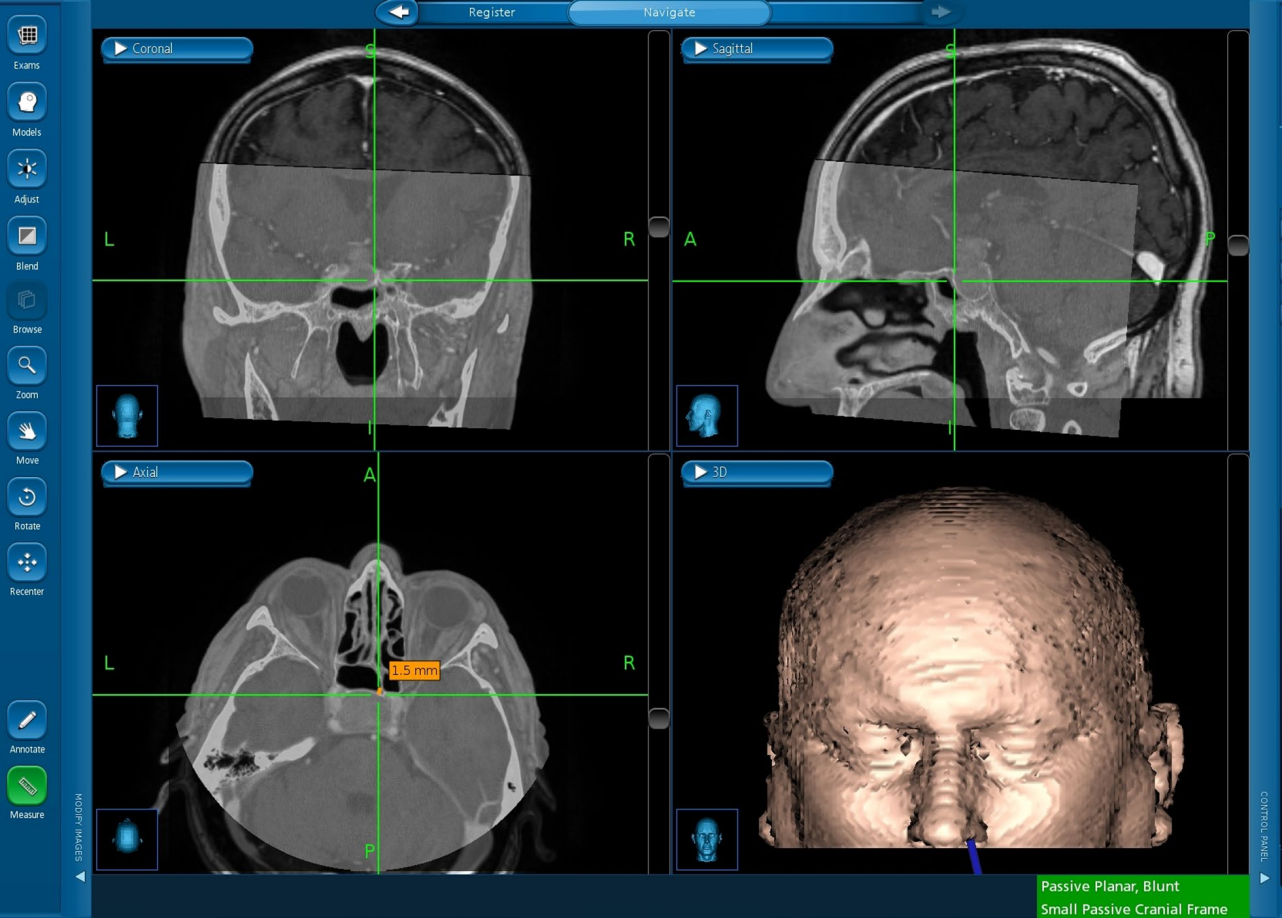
Example of measurement of the distance between pointer’s tip and bony structure (intrasphenoidal septum in this case) on the screen of the neuronavigation system in a presurgical CT scan
